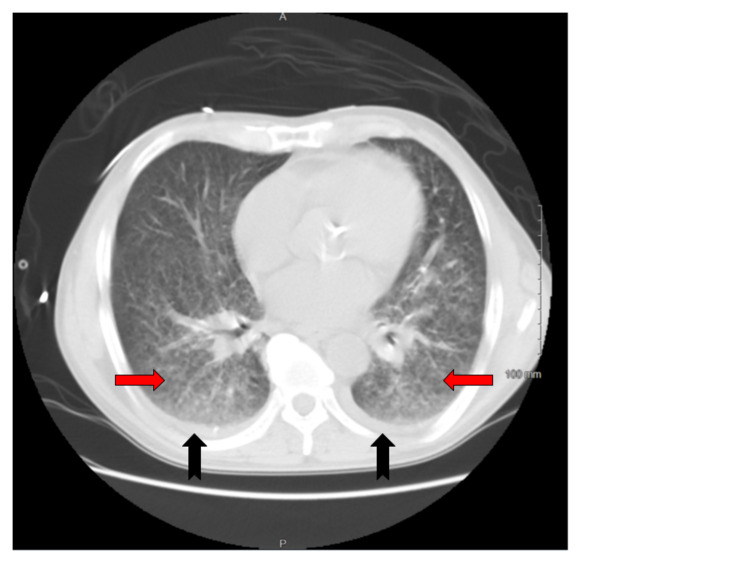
CT scan of the chest (axial view, lung window) at the level of the heart showing extensive, diffuse bilateral ground glass opacities (red arrow) without area of predominant consolidation and bilateral trace pleural effusion (black arrow), consistent with acute histoplasmosis in the patient with HIV.
